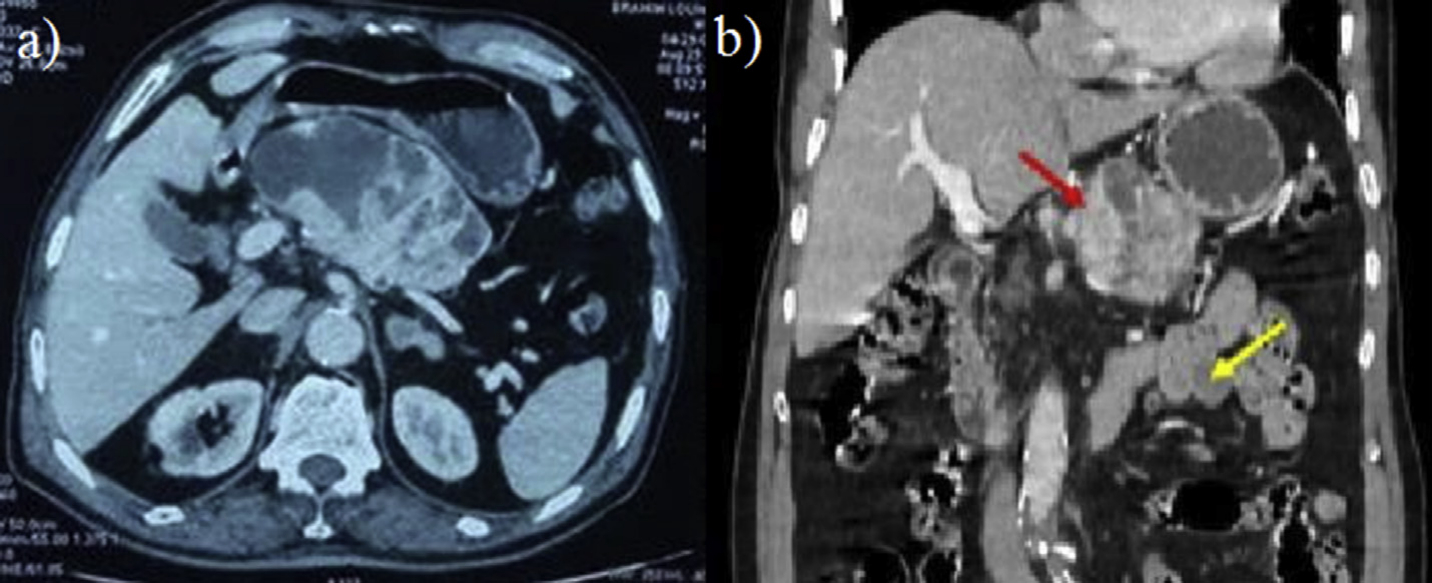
CT abdomen: (a) axial section showing the pancreatic tumor, (b) coronal section showing the pancreatic tumor (red arrow) and the jejunal GIST (yellow arrow). (For interpretation of the references to colour in this figure legend, the reader is referred to the Web version of this article.)
